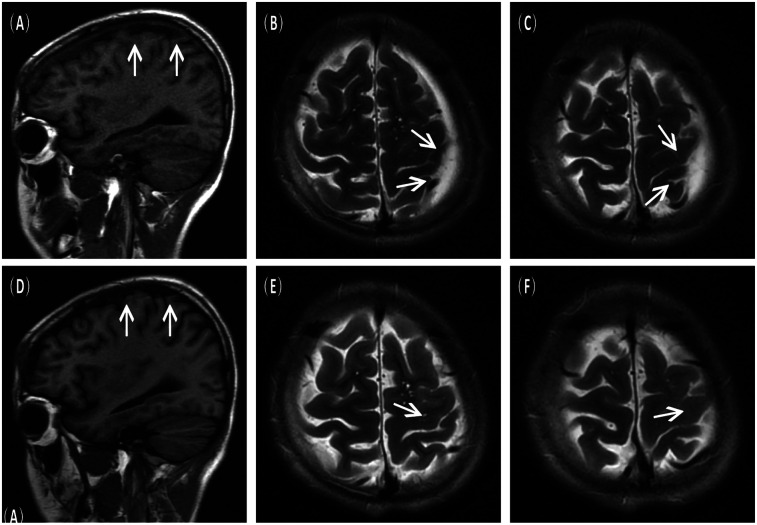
Postoperative imaging findings. (A–C) Immediate postoperative MRI scans showing resolution of the subdural hematoma and decreased size of the arachnoid cyst. (D–F) Follow–up MRI scans at 6 months post–surgery confirmed no recurrence of the cyst.
